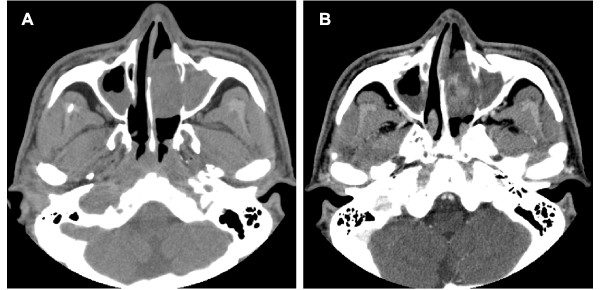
Computed tomographic images before surgery. (A) Non-contrast-enhanced PNS CT scan showing the primary lesion in the left nasal cavity. (B) The lesion showed heterogeneous enhancement on contrast-enhanced CT images.
